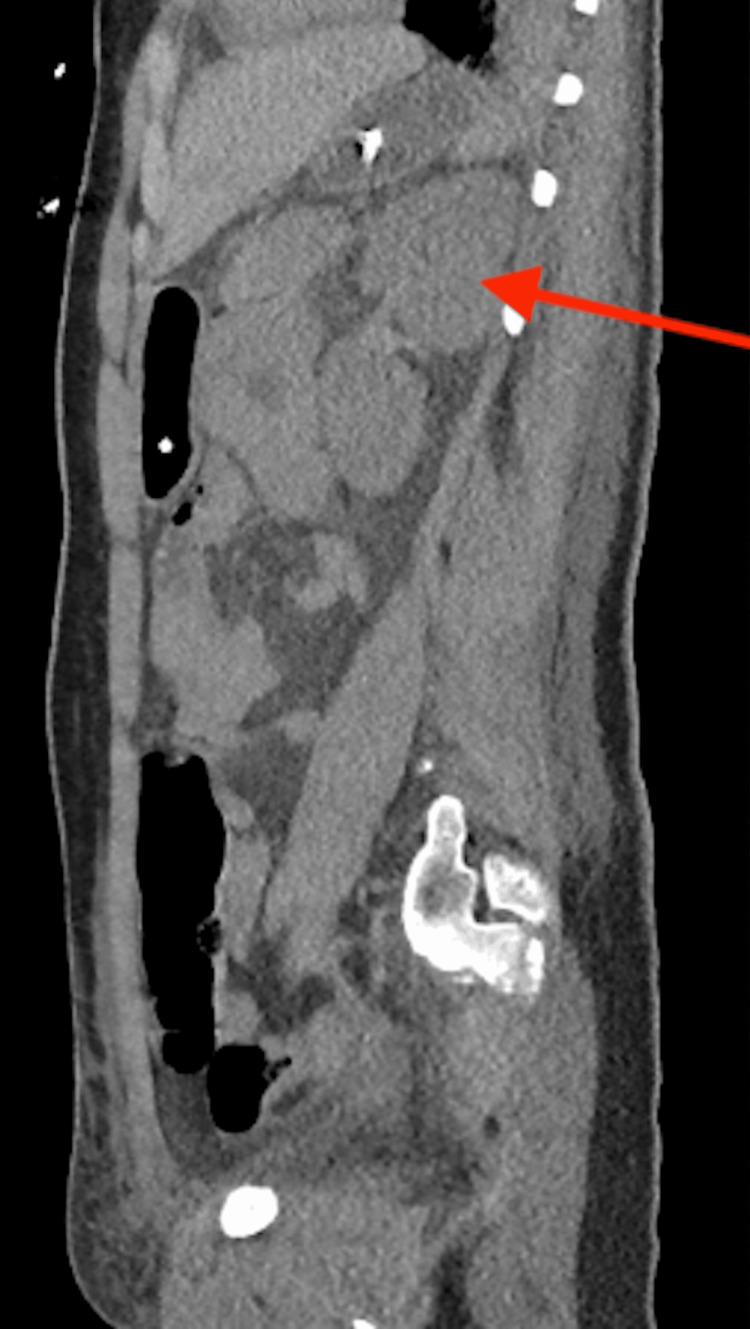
Left suprarenal mass on CT abdomen and pelvis The arrow shows the left suprarenal mass. CT, computed tomography
